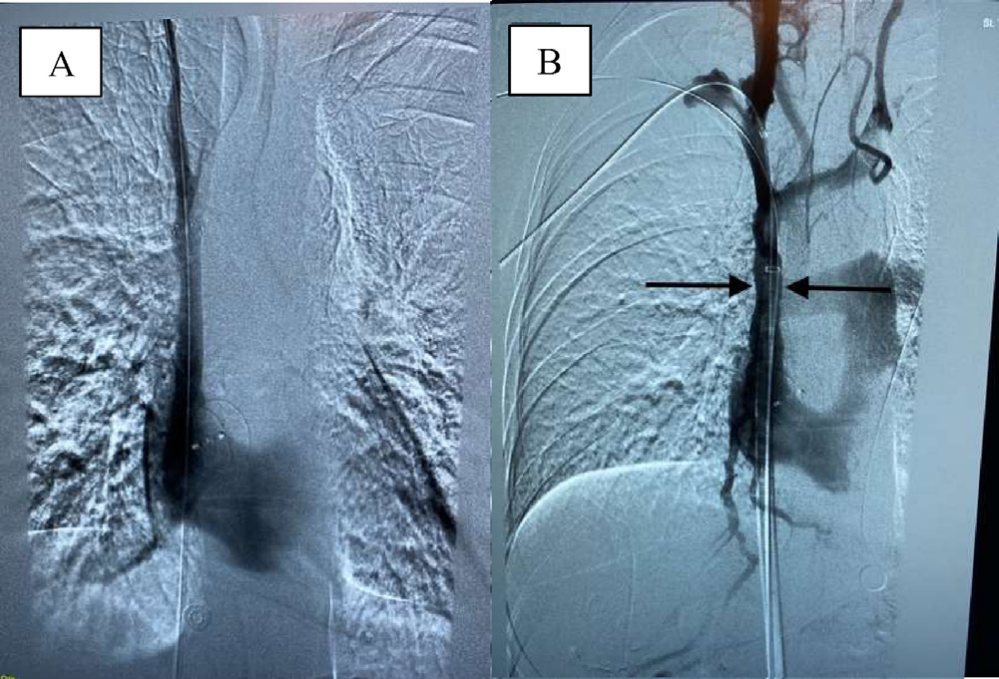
X-ray venography showing a non-obstructed SVC post-thrombectomy. A. Restoration of blood flow in the superior vena cava. B. Placement of a stent to prevent residual stenosis of the SVC.
